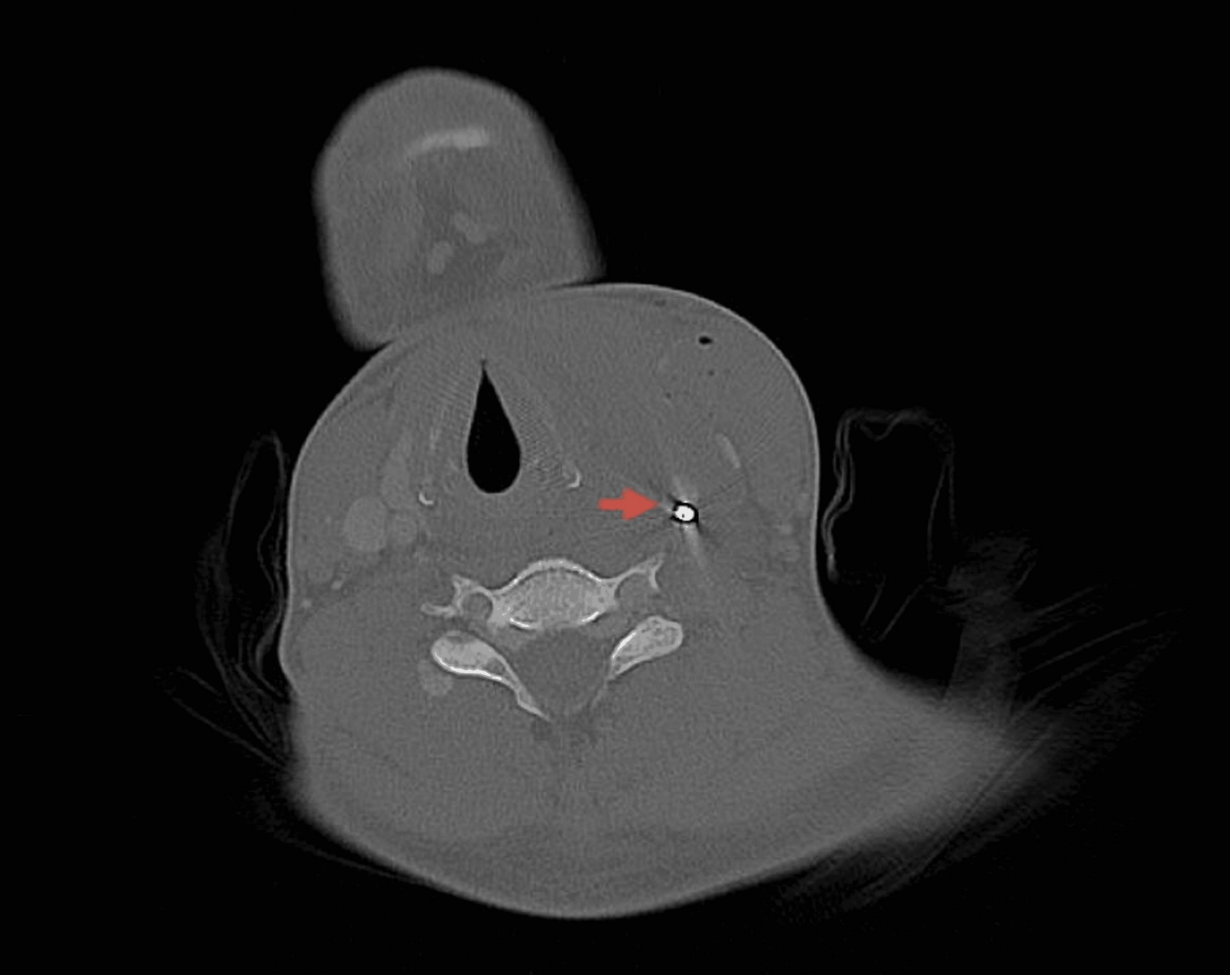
Preoperative CT. Left metallic foreign body with metallic artifact very close and abutting to the common carotid artery.
